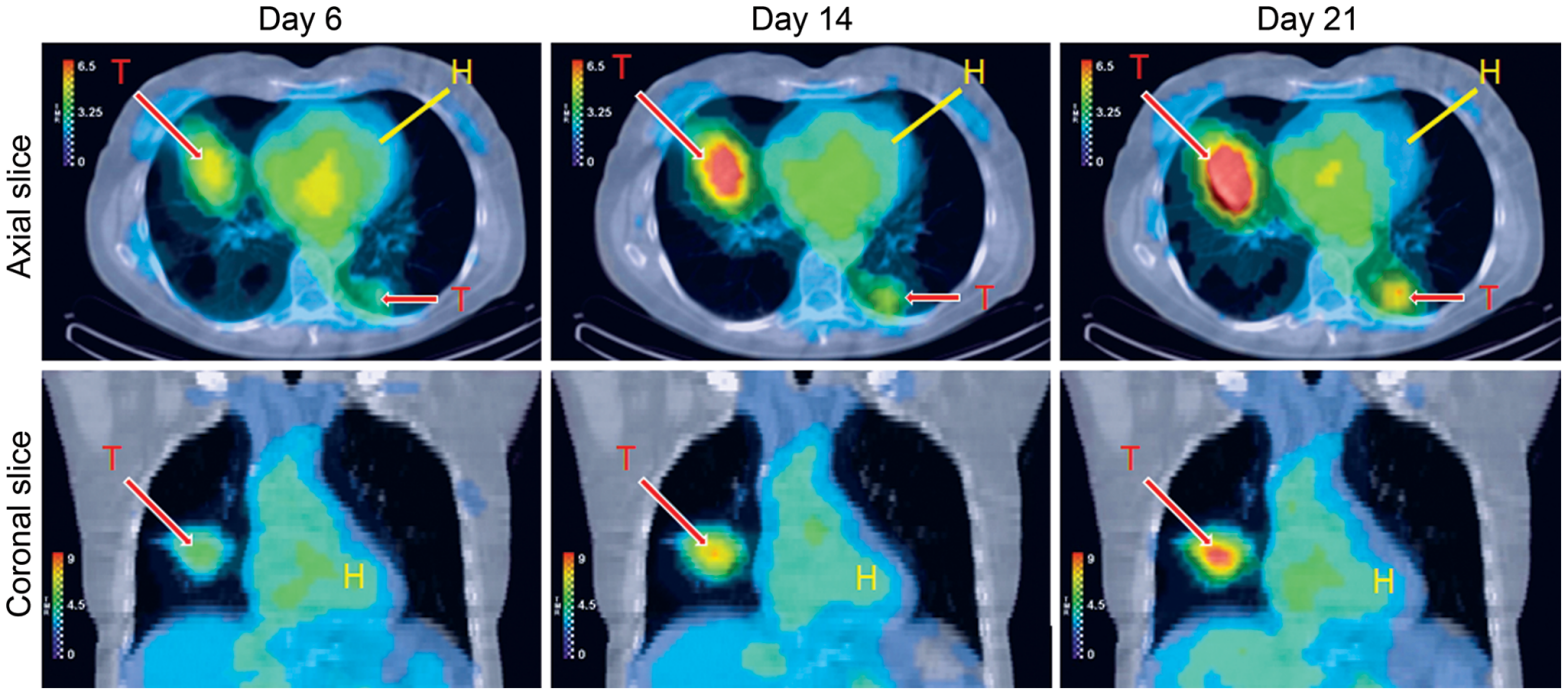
^131^I-CLR1404 accumulates in a patient with metastatic colon cancer. As part of a phase I clinical trial, SPECT images were acquired between 6 and 21 days after administration of ^131^I-CLR1404. Areas of increased signal intensity corresponded to known areas of metastatic colon cancer in both lungs. Prolonged retention of this agent within these lesions was also demonstrated. T, tumor; H. heart.
